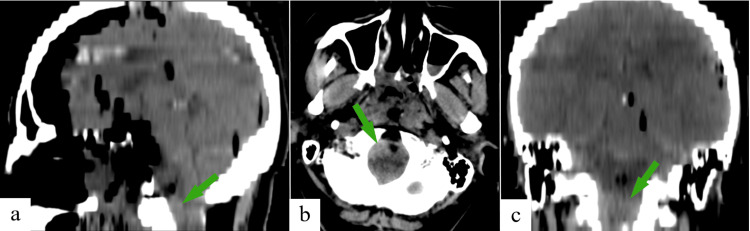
CT scan of the brain immediately after surgery. Postoperative pneumocephalus, subtotal tumor removal: (a) sagittal plane; (b) axial plane; (c) coronal plane (the arrows indicate the residual tumor fragment at the level of C1-C2). CT, computed tomography
